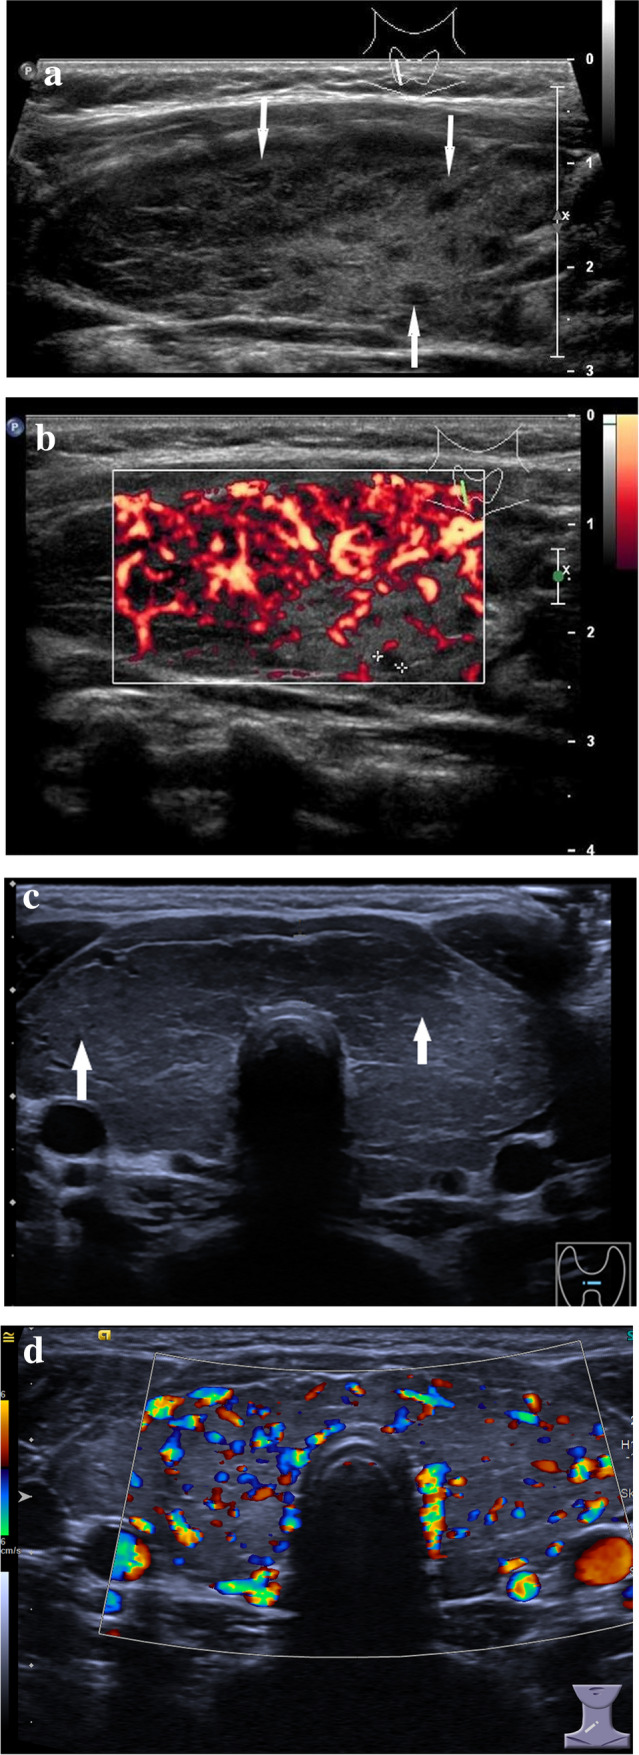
Conventional US and color Doppler US images of diffuse thyroid disease. a B-mode US image in a longitudinal plane shows enlarged thyroid with diffuse spreading of hypoechoic lesions (arrows) in a 17-year-old girl with Hashimoto thyroiditis. b Color Doppler US image in a longitudinal plane shows increased vascularity in a 17-year-old girl with Hashimoto thyroiditis. c B-mode US image in a transverse plane of an enlarged thyroid with hypoechoic areas (arrows) in an 11-year-old girl with Graves’ disease. d Color Doppler US image in a transverse plane shows increased vascularity of the thyroid gland in an 11-year-old girl with Graves’ disease referred to as “thyroid inferno”
